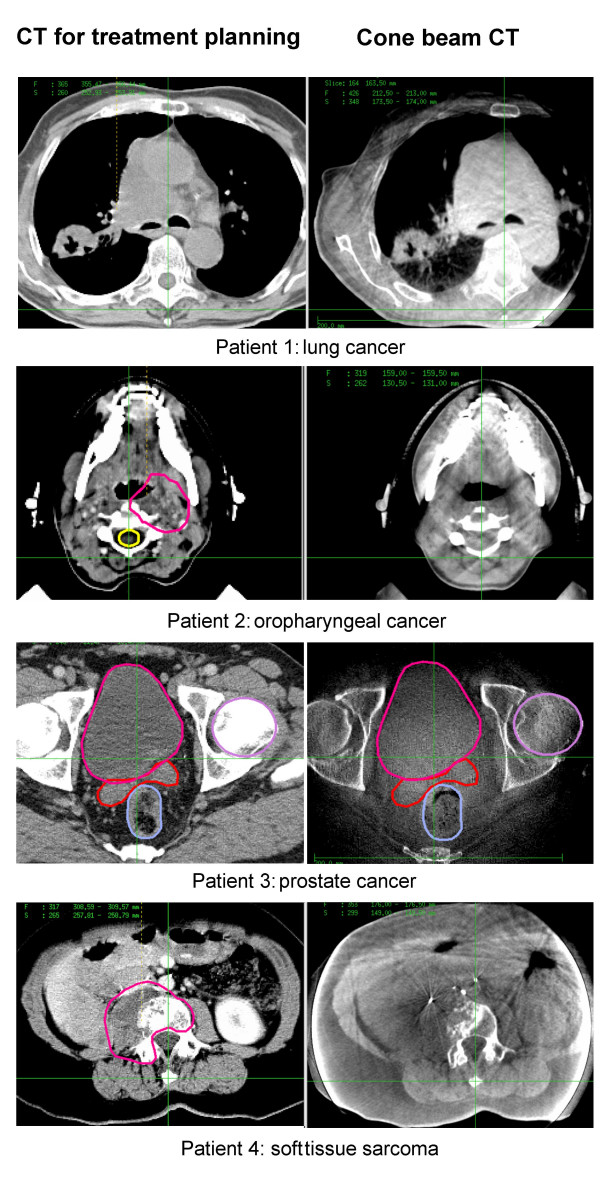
Clinical examples of cone beam (right side) compared to diagnostic treatment planning CT (left side).
